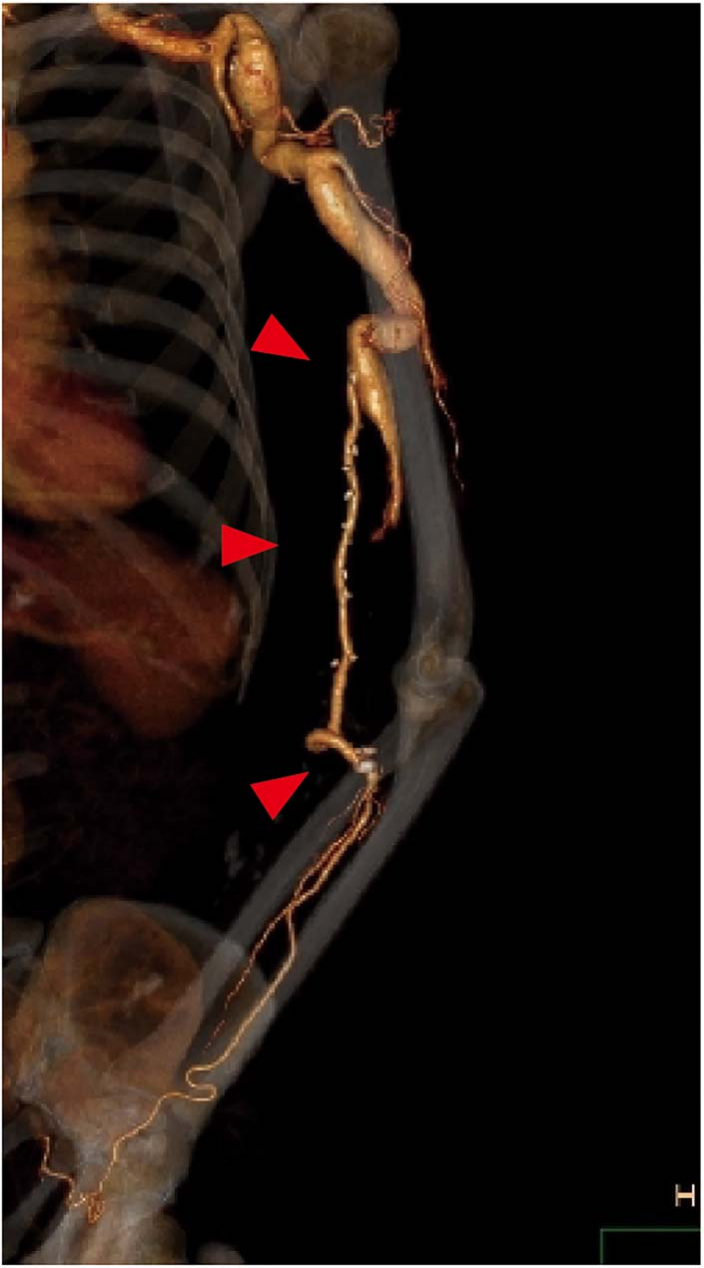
Postoperative contrast CT showing the patent great saphenous vein graft (red arrows) anastomosed from the brachial to ulnar artery. To prevent graft occlusion due to elbow flexion, the graft was routed through the ulnar side of the elbow.
